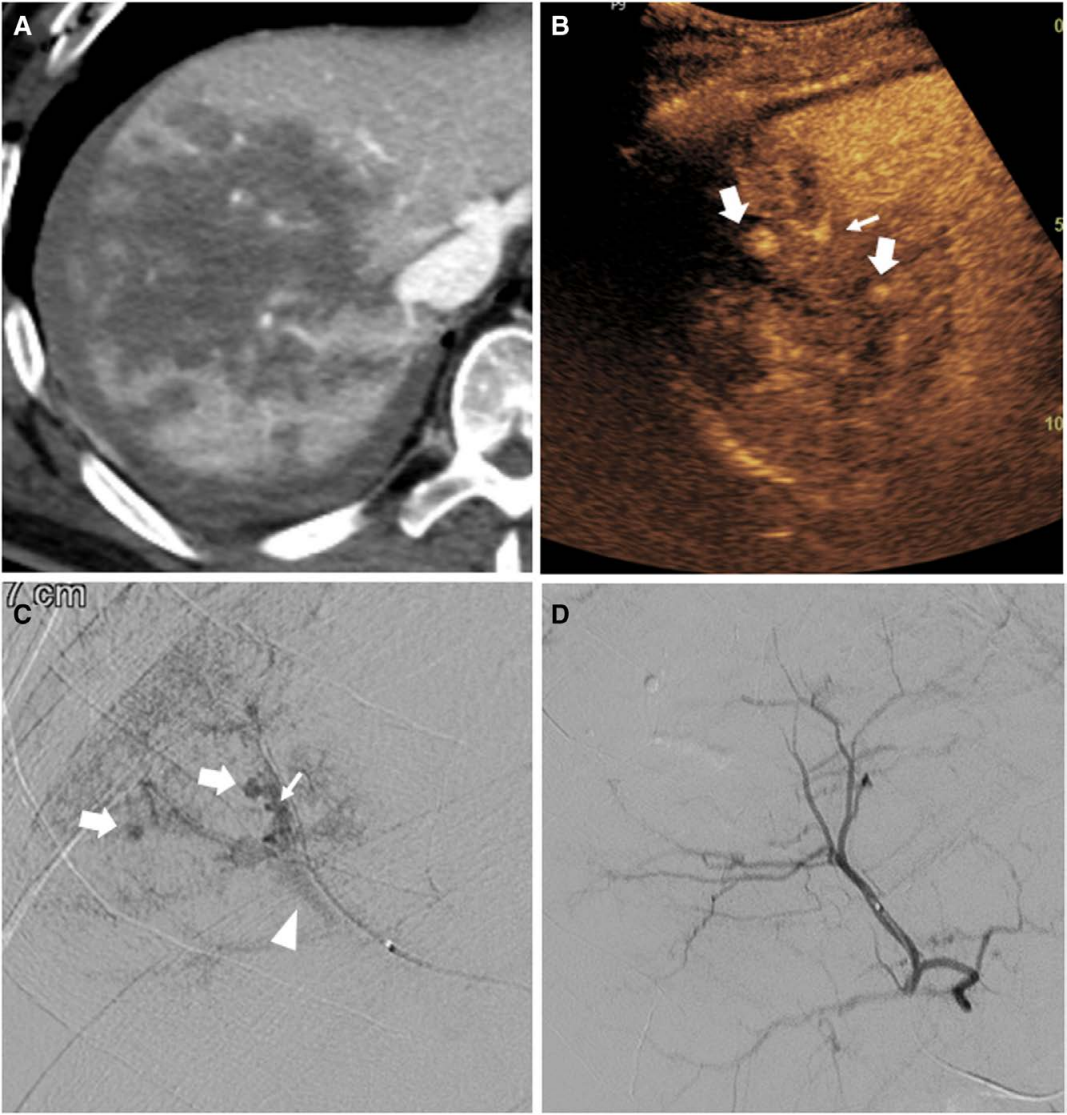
Liver capsular vascular injury combined with bare area hematoma in a 73-year-old male after a motor vehicle accident. (A) Contrast-enhanced axial CT scan reveals a large non-enhancing lesion in the right liver and multiple enhancing foci; however, it is unclear whether it is vascular injury requiring angioembolization or partial volume artifacts of normal vascular structure. (B) The subsequent CEUS demonstrates the vascular injury as hyperechoic round spots (thick arrow) and fountain-like hyperechoic lesions with long-tail signs (thin arrow), suggesting pseudoaneurysms and active bleeding. (C) Given the CEUS finding, selective angiogram of the right hepatic artery is performed, which demonstrates multiple pseudoaneurysms (thick arrow) and active bleeding (thin arrow), and early drainage of the hepatic vein suggesting arteriovenous fistula (arrowhead). (D) Complete total embolization of the right hepatic artery was performed using glue, and a subsequent angiography revealed successful complete obliteration of the right hepatic artery. In this case, CEUS could accurately diagnose the site of trauma-related vascular injury and guide the subsequent embolization procedure. CEUS = contrast-enhanced ultrasound, CT = computed tomography.
